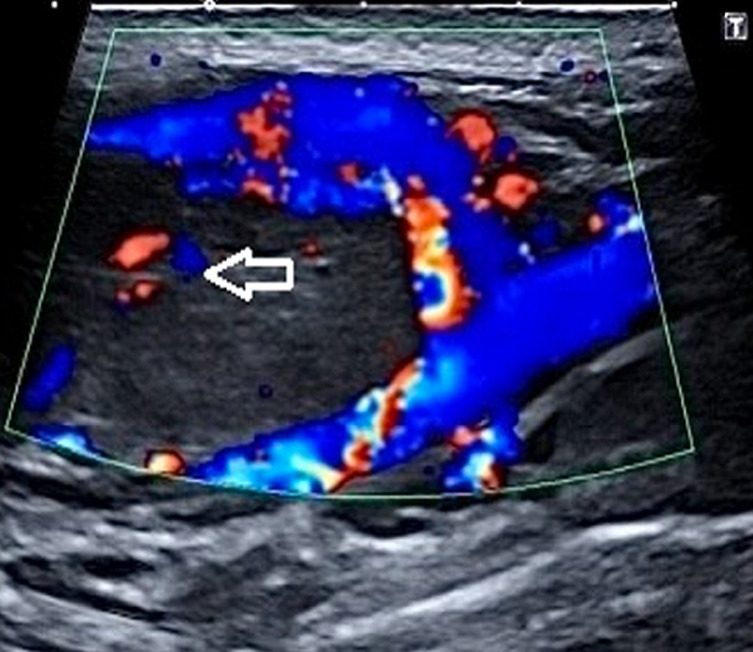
minimal vascularity within the lesion on color Doppler (arrow)
